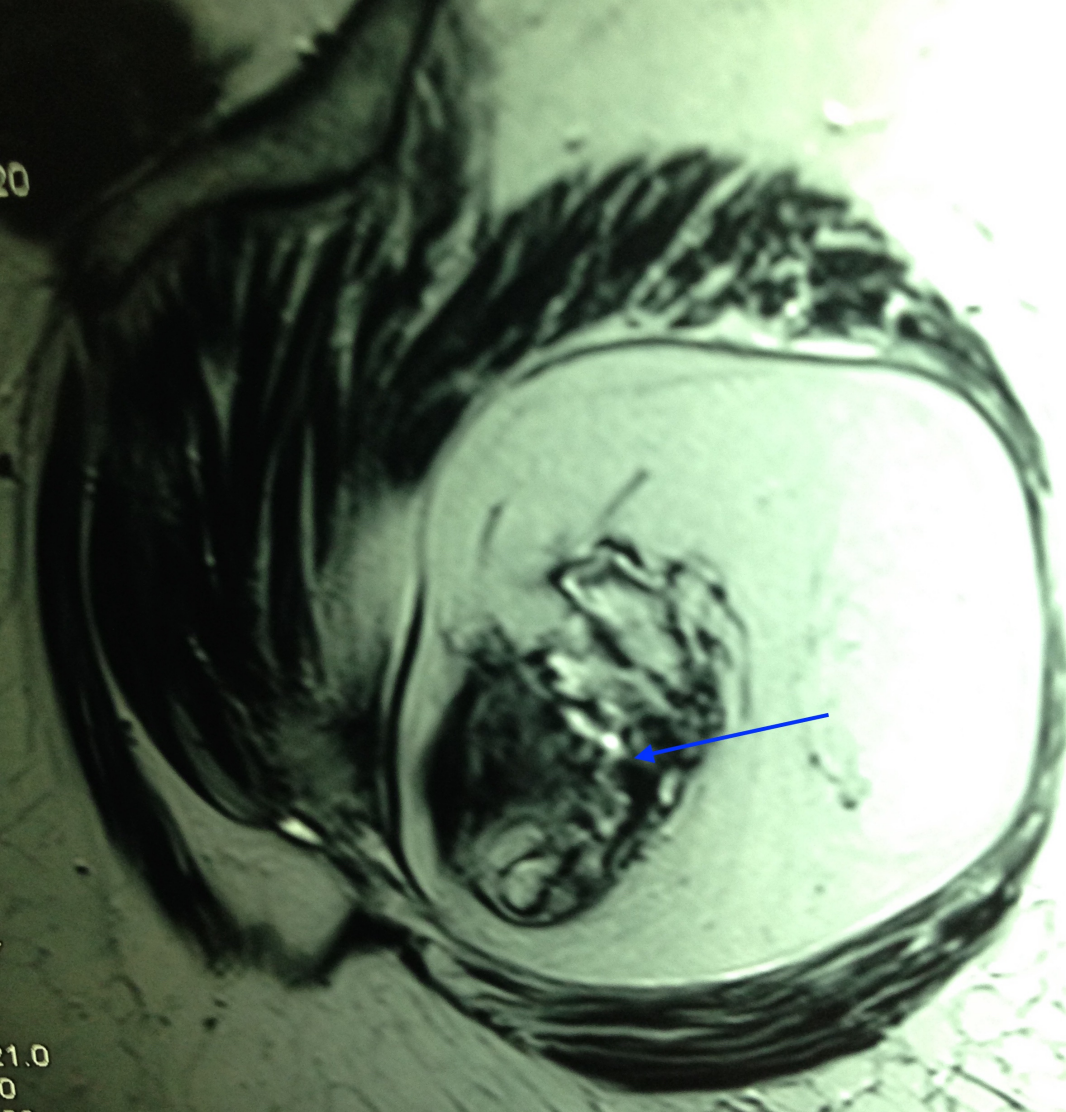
T1-weighted MRI showing tumour mass
